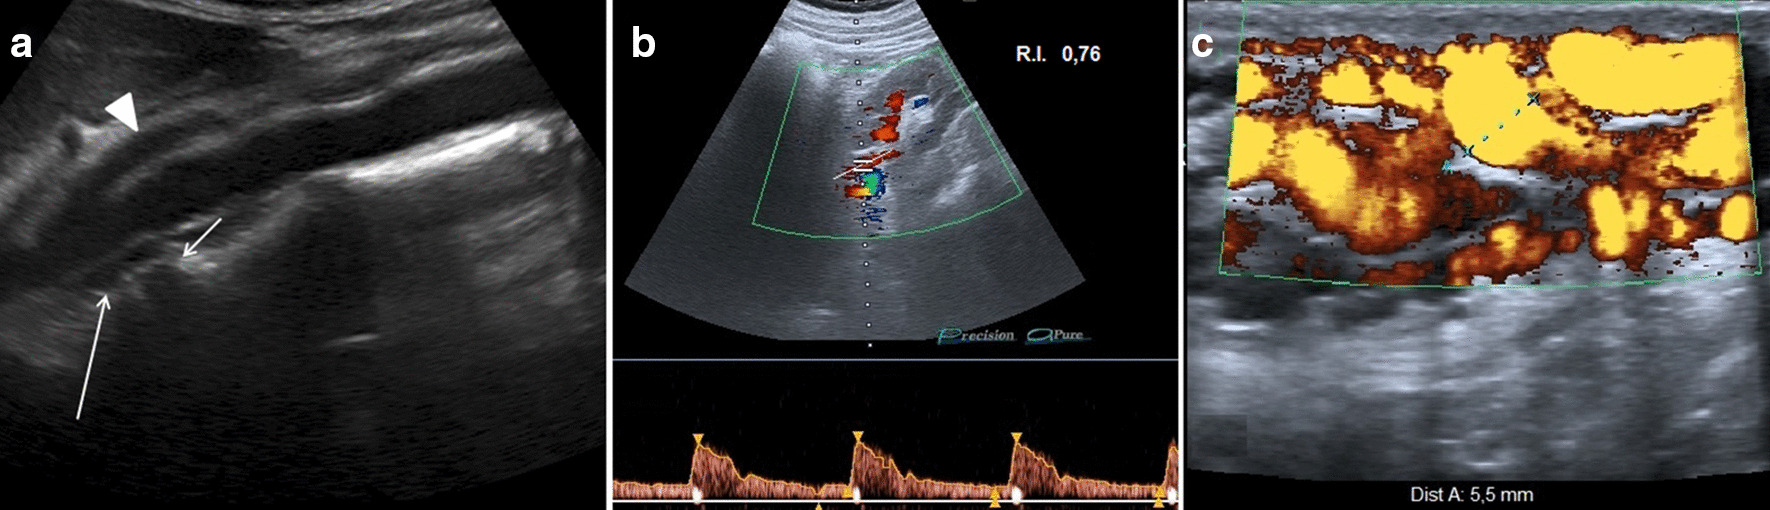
a B-mode US: longitudinal sub-xyphoid scan of the abdominal aorta at the level of the arising superior mesenteric artery (arrowhead), showing the retro-aortic left renal vein (long arrow) compressed between the abdominal aorta (*) and an osteophyte (short arrow) of the third lumbar vertebral body. b Duplex Doppler US of the left renal artery showing a higher resistive index value (0.76). c Power Doppler US of the pampiniform plexus showing left varicocele (grade IV on Sarteschi’s scale)
